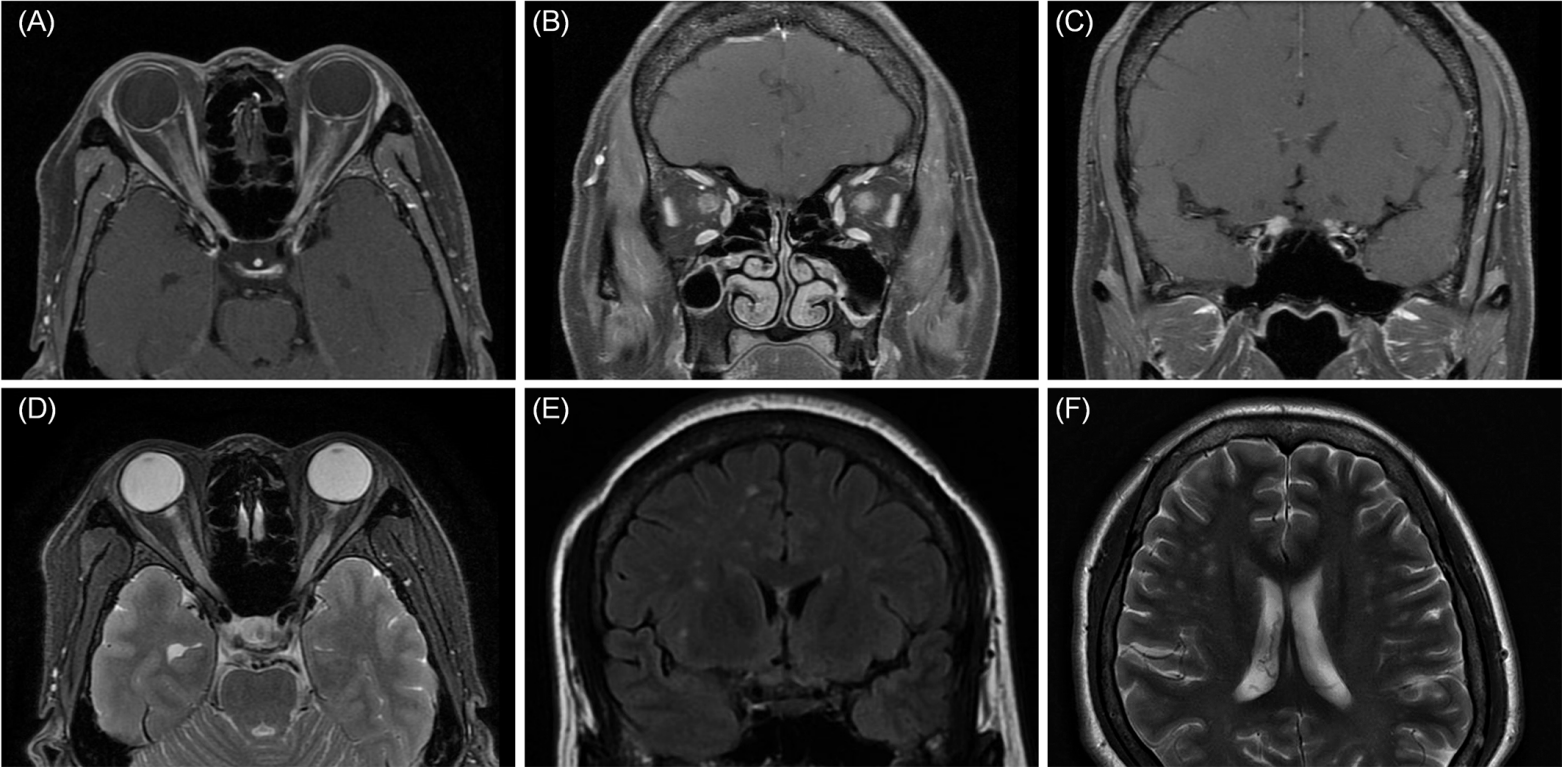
MRI axial (A) and coronal (B, C) showed bilateral enhancement of the optic nerve sheath, optic nerve, and chiasm. (D) Fat-suppressed T2-weighted sequences showed a hypersignal of the bilateral optic nerves and sheath. (E, F) Coronal and axial MRI was found to have multifocal patchy lesions in white matter of frontal cortex and the periventricular white matter.
